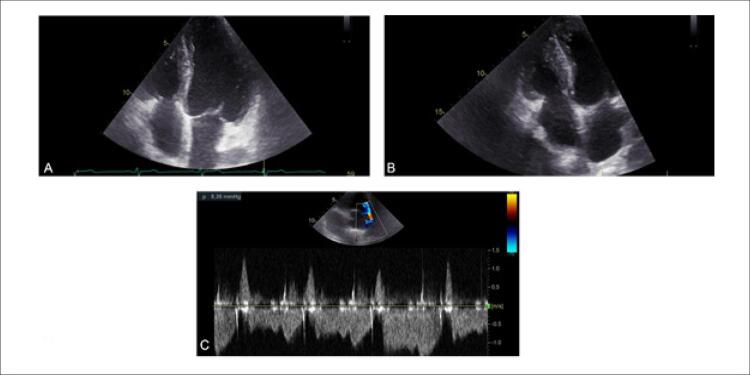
Ecocardiografia transesofágica e transtorácica bidimensional. A) corte apical de quatro câmaras na sístole mostrando a válvula tricúspide aberta e retraída e a válvula mitral fechada; B) corte apical de quatro câmaras na sístole (foco no ventrículo direito) mostrando a válvula tricúspide aberta e retraída e a válvula mitral fechada; C) curva do fluxo tricúspide no Doppler contínuo, mostrando uma equalização da pressão entre o átrio direito e o ventrículo direito.
